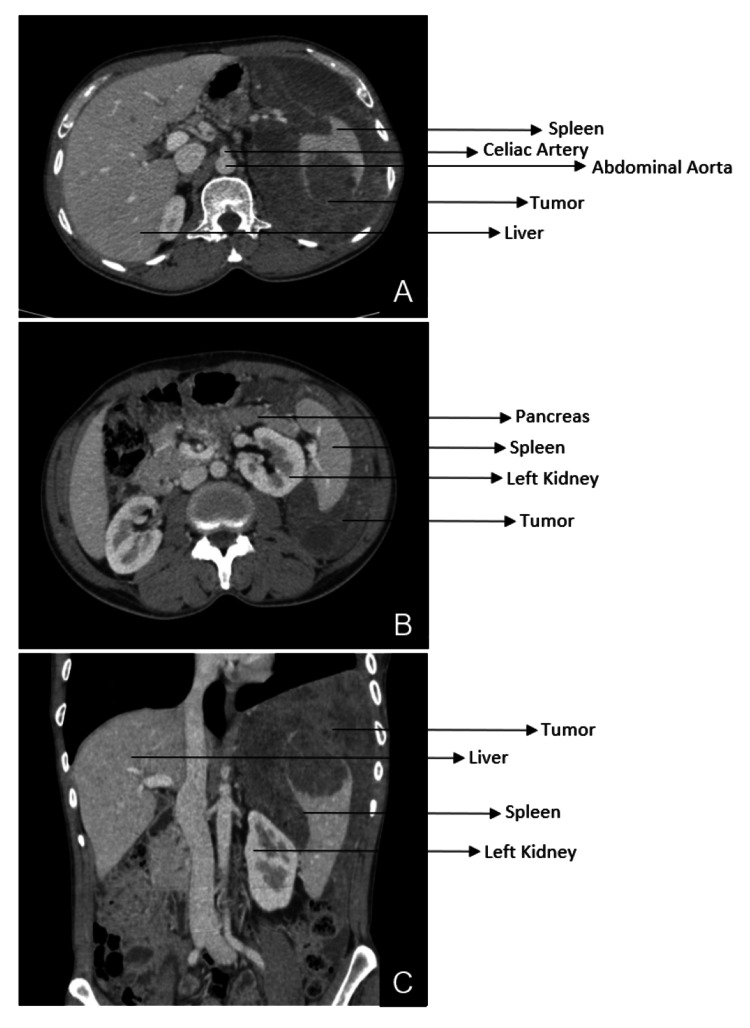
Preoperative CT scan of the thorax and abdomen with contrast (A) Axial section showing left infra-diaphragmatic soft tissue mass encasing the spleen; (B) Axial section showing left infra-diaphragmatic soft tissue mass displacing the left kidney anteromedially; (C) Coronal section showing infra-diaphragmatic extension of the tumor
